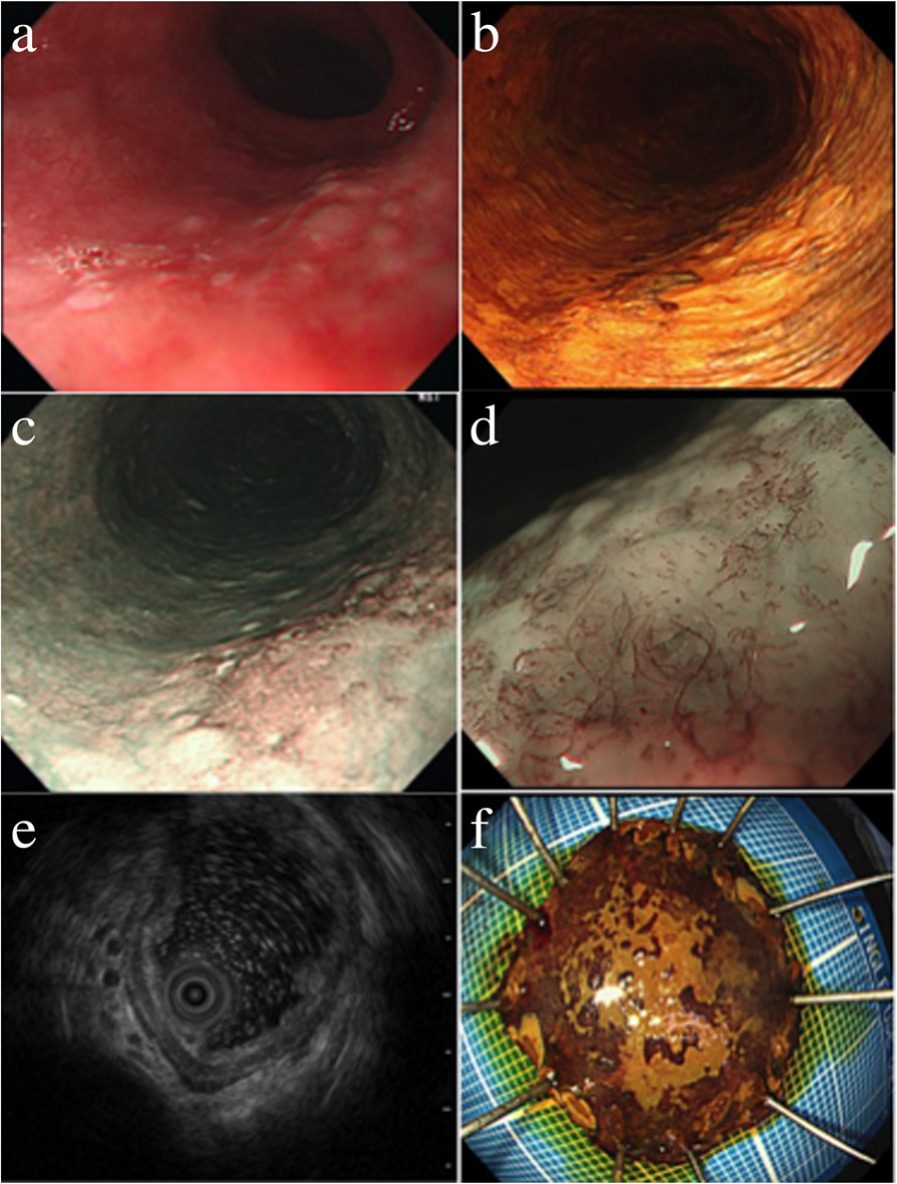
a Esophagogastroduodenoscopy shows an irregular, reddish, flat lesion in the posterior wall of the mid-esophagus. b Lugol’s iodine chromoendoscopy shows an unstained lesion that is located in the posterior wall of the mid-esophagus. c Narrow band imaging endoscopy shows a brownish area. d Magnifying endoscopy with narrow band imaging shows a microvascular pattern with irregular, fine, reticular blood vessels near the center of the lesion. e Endoscopic ultrasound image of the lesion limited to the mucosa. f En bloc resection by endoscopic submucosal dissection
